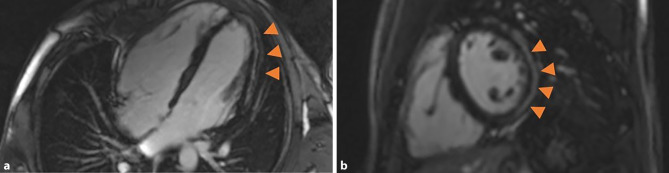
Cardiac magnetic resonance imaging. Four-chamber view (a) and short-axis view (b) demonstrate right ventricular dilation and subepicardial late gadolinium enhancement (arrows) involving the mid-apical inferolateral and anterolateral walls
